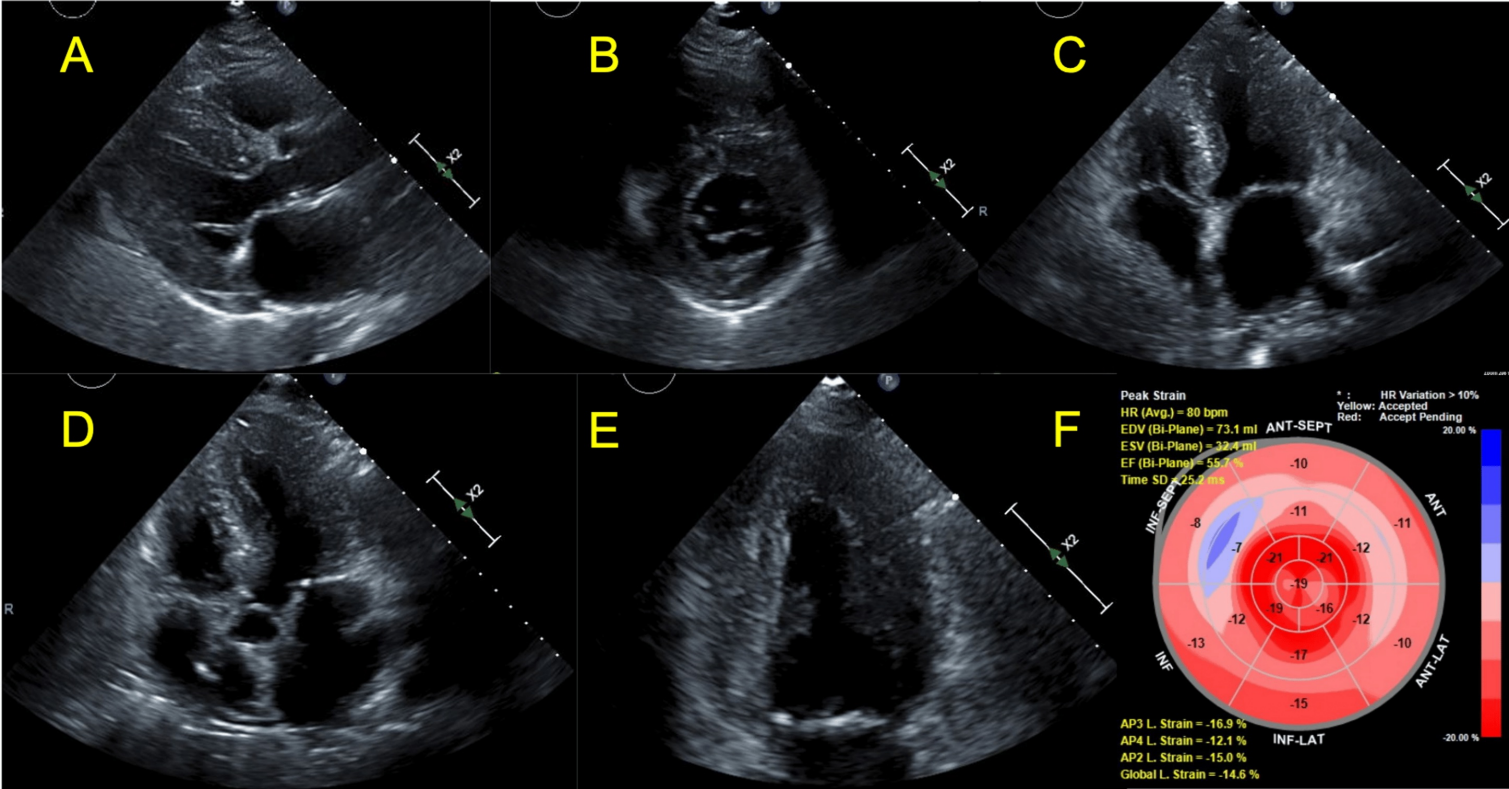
(A) Parasternal long-axis view showing thickened left ventricular septum and inferior wall. (B) Parasternal short-axis view at the level of the mitral valve showing concentric thickening of the left ventricle. (C) Apical four-chamber view showing thickening of the inferoseptal and lateral walls. (D) Apical five-chamber view showing thickening of the anteroseptal and inferolateral walls. (E) Apical two-chamber view showing thickening of the anterior and inferior walls. (F) Global longitudinal strain is reduced at -14.6% (normal is negative values greater than 18%). Strain values are relatively more normal at the apex (“apical sparing”). These findings are typical of cardiac amyloidosis
